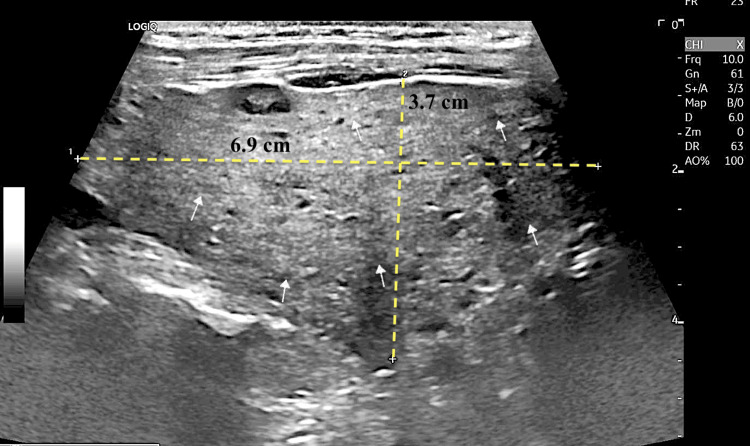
Thyroid ultrasound showing heterogeneous echotexture (white arrows) throughout the right thyroid lobe parenchyma The thyroid is enlarged, with generalized parenchymal heterogeneity, suggestive of diffuse thyroid disease. The right thyroid lobe measures 6.9 × 3.7 cm (yellow dotted lines).
